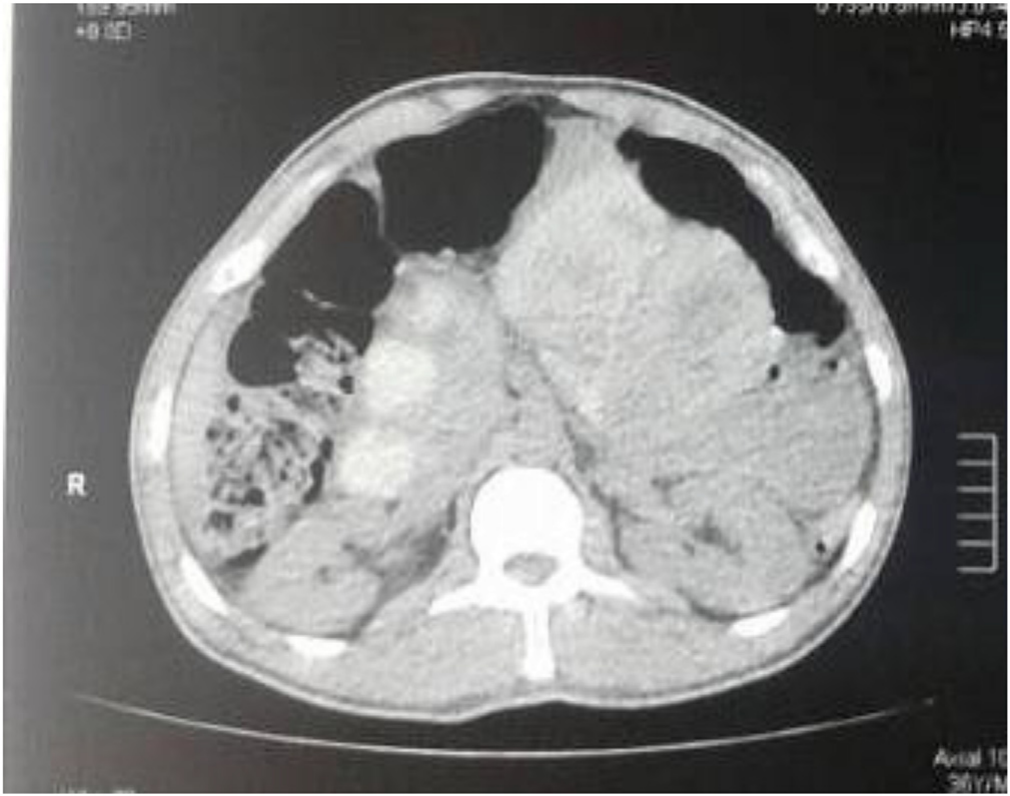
CT scan of the abdomen showing dilated bowel loops and inflammatory mass on the right iliac fossa.
